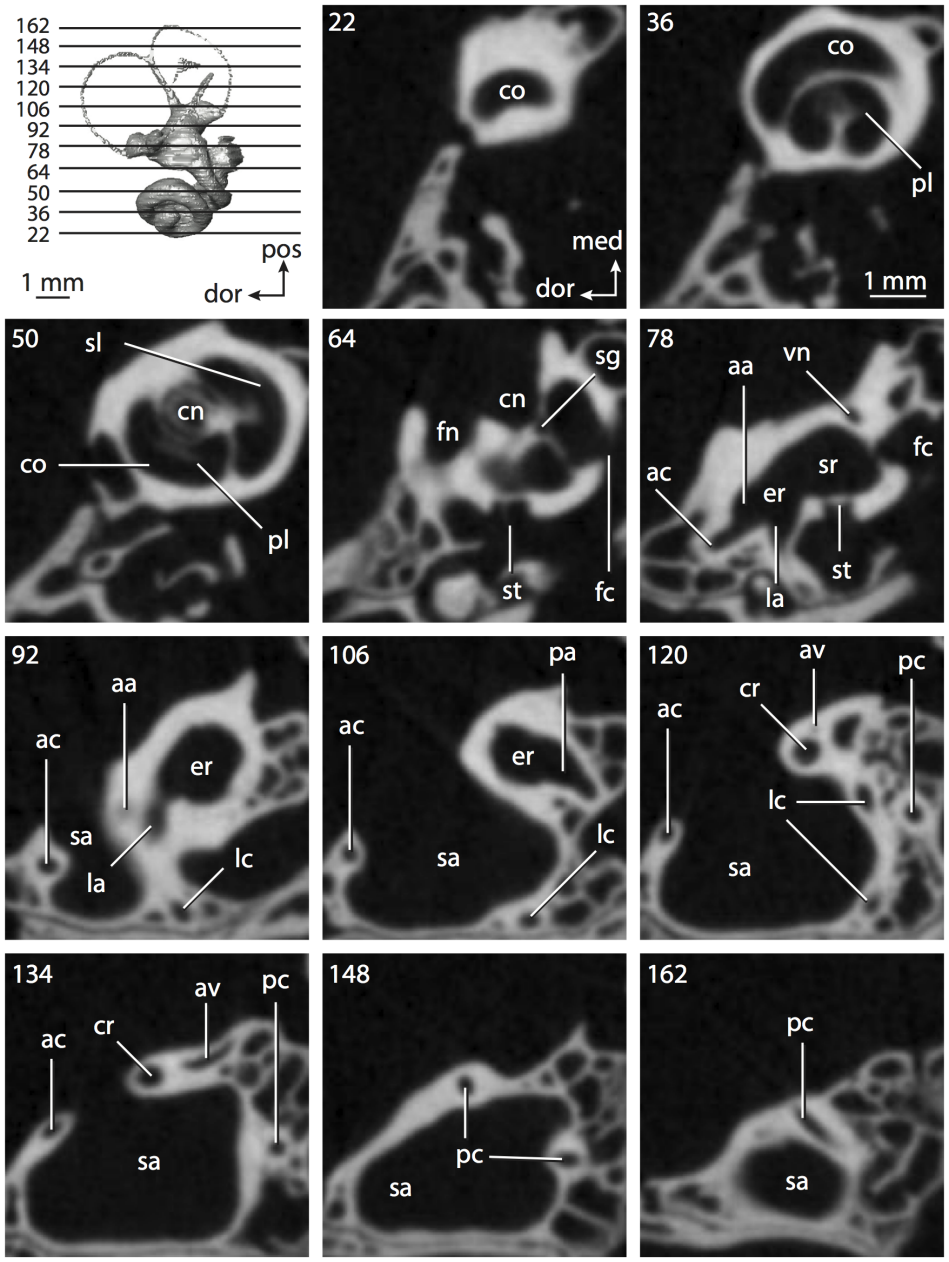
CT slices through ear region of*Pteropus lylei*. Abbreviations listed at the end of the Materials and Methods section.
